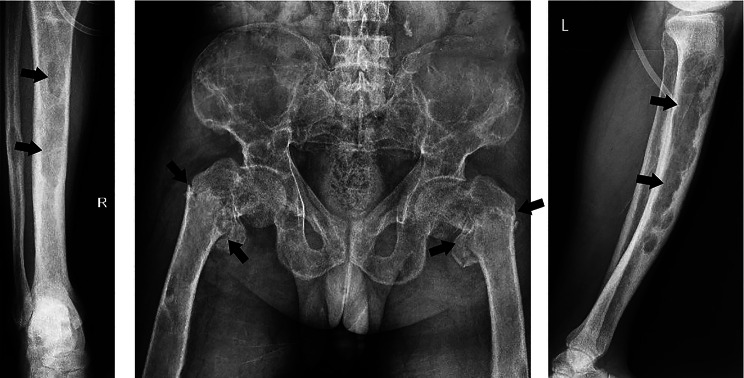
Pelvic and lower leg X-ray images; bilateral fractures in the intertrochanteric region of femur and diffused lytic lesions with mild to moderate expansion mainly in medullary bone
